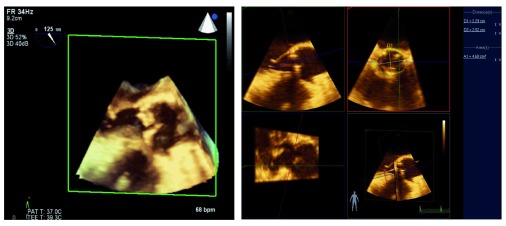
The three-dimensional view of the left ventricular outflow tract (left) allows precise measurement of the aortic valve annulus (right). This technique is used to guide percutaneous aortic valve replacement and has been shown to be equivalent to computed tomography angiography for this purpose. 3D, three-dimensional; bpm, beats per minute; PAT T, patient temperature; TEE T, transesophageal echocardiography temperature.
